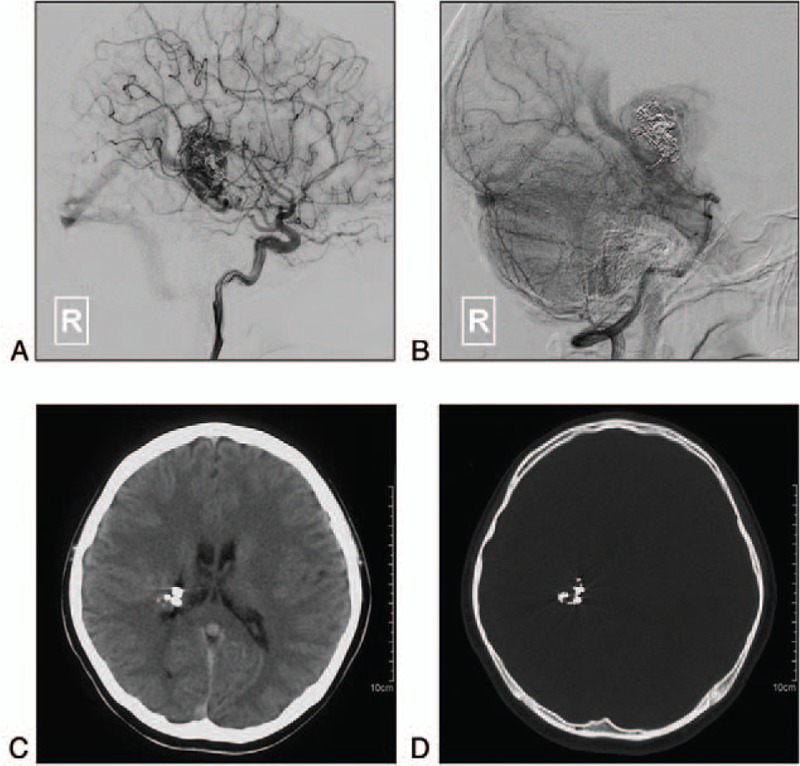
A-B: Follow-up DSA of the right ICA (A) and VA (B) 9 months later shows the residual BAVM nidus and the disappearance of the varix. C-D: CT shows the disappearance of the occupying lesion and perilesional edema. BAVM = brain arteriovenous malformation, CT = computed tomography, DSA = digital subtraction angiography, ICA = internal carotid artery, VA = vertebral artery.
